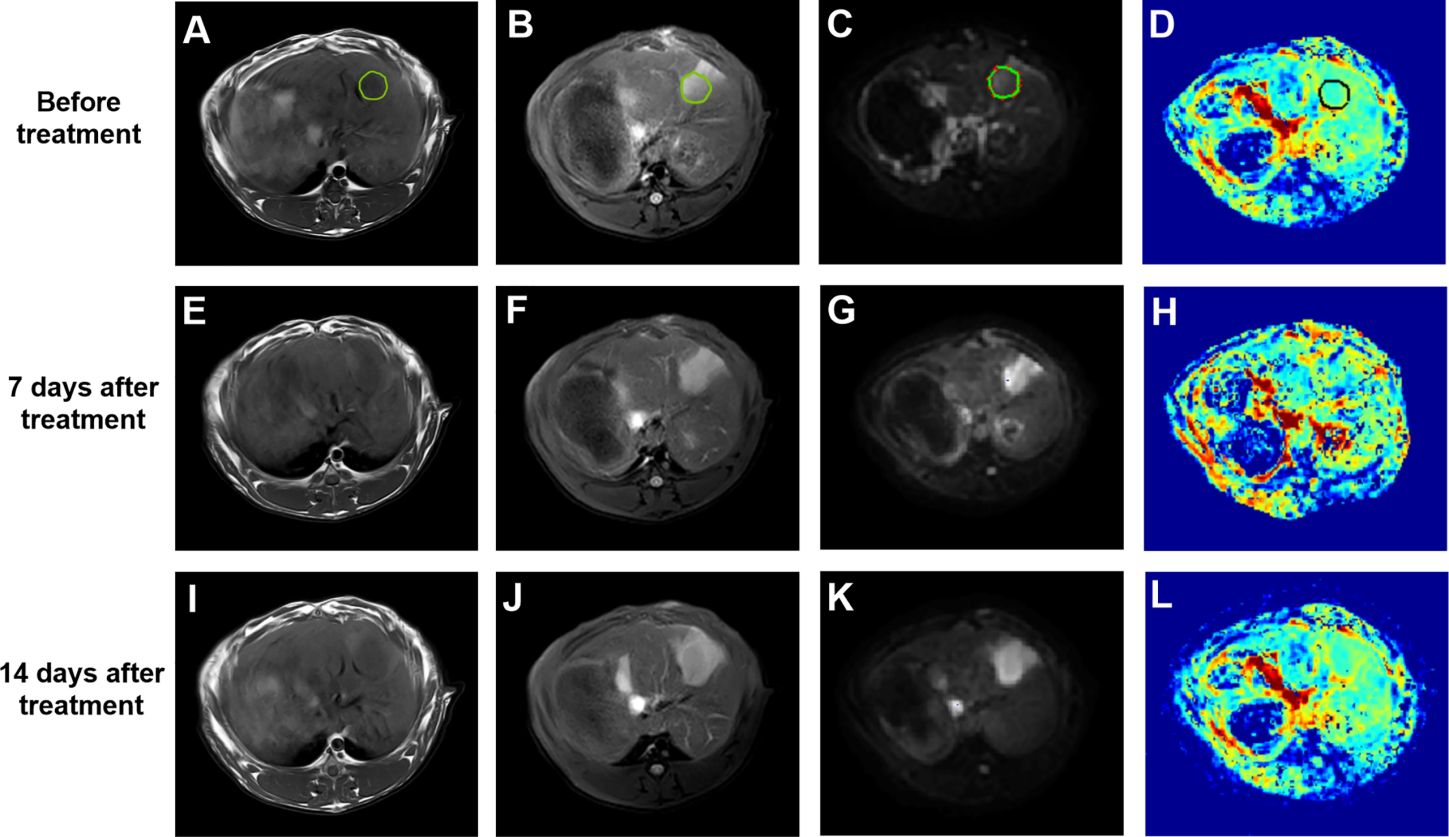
(A–D) T1WI, T2WI, DWI (b=500 s/mm^2^) and pseudo-color image of D before treatment, the green or black circle is the area where the tumor was planted. (E–H) T1WI, T2WI, DWI (b=500 s/mm^2^) and pseudo-color image of D 7 days after treatment. (I–L) T1WI, T2WI, DWI (b=500 s/mm^2^) and pseudo-color image of D 14 days after treatment. DWI, diffusion-weighted imaging; D, true diffusion coefficient.
